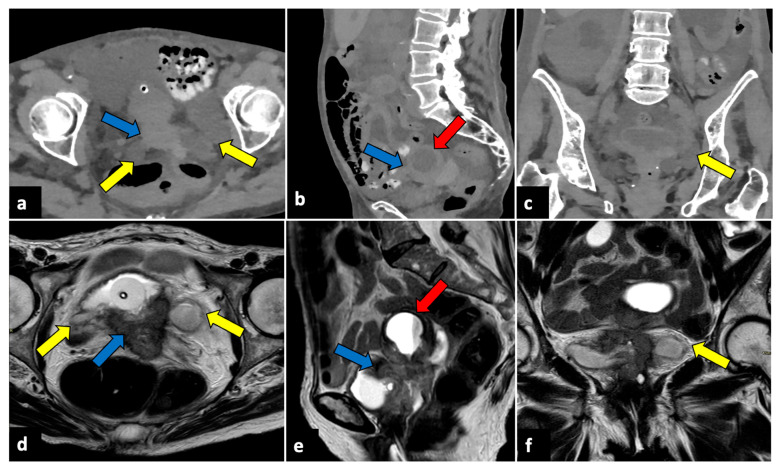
Advanced uterine cervical cancer in a 58-year-old subject attending the emergency department for abdominal pain and haematuria. CT axial (a), sagittal (b), and coronal (c) non-contrast images reveal the presence of a pelvic mass (blue arrows) and ureter causing ureteral obstruction (yellow arrows). The patient further underwent an MRI examination (d–f), which demonstrated a cervical tumour (blue arrows) invading the parametrium and ureters bilaterally (yellow arrows), the vagina, and the bladder. The uterine cavity was also obstructed and dilatated (red arrows).
